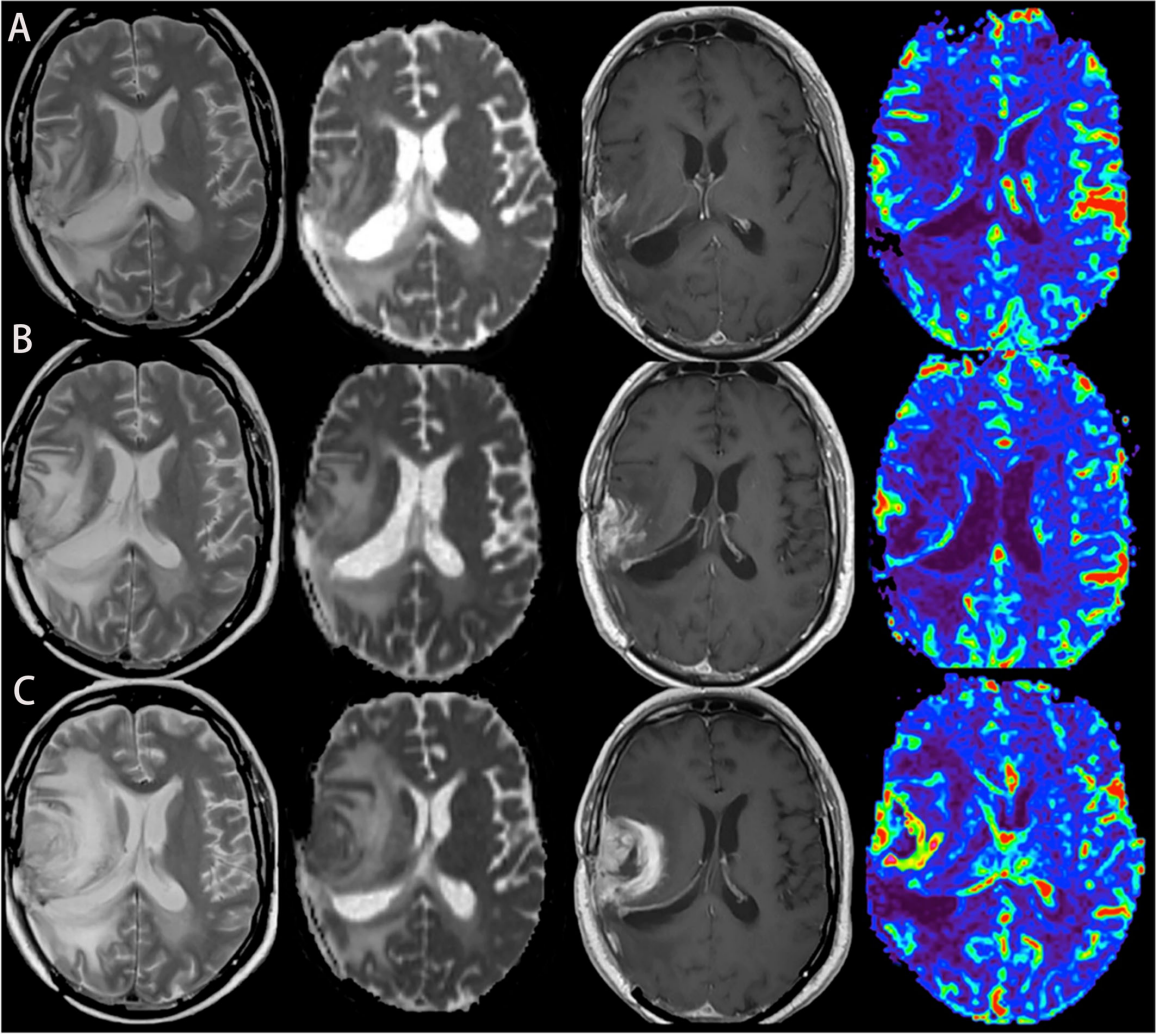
A case of glioblastoma relapsed during immunotherapy, T2, ADC map, T1-enhanced, and CBV map from left to right. (A–C) MRI was performed in the 2nd, 6th, and 8th months of immunotherapy, respectively, showing that the edema degree of the lesion was gradually aggravated, the enhancement was more obvious, and the perfusion was higher.
